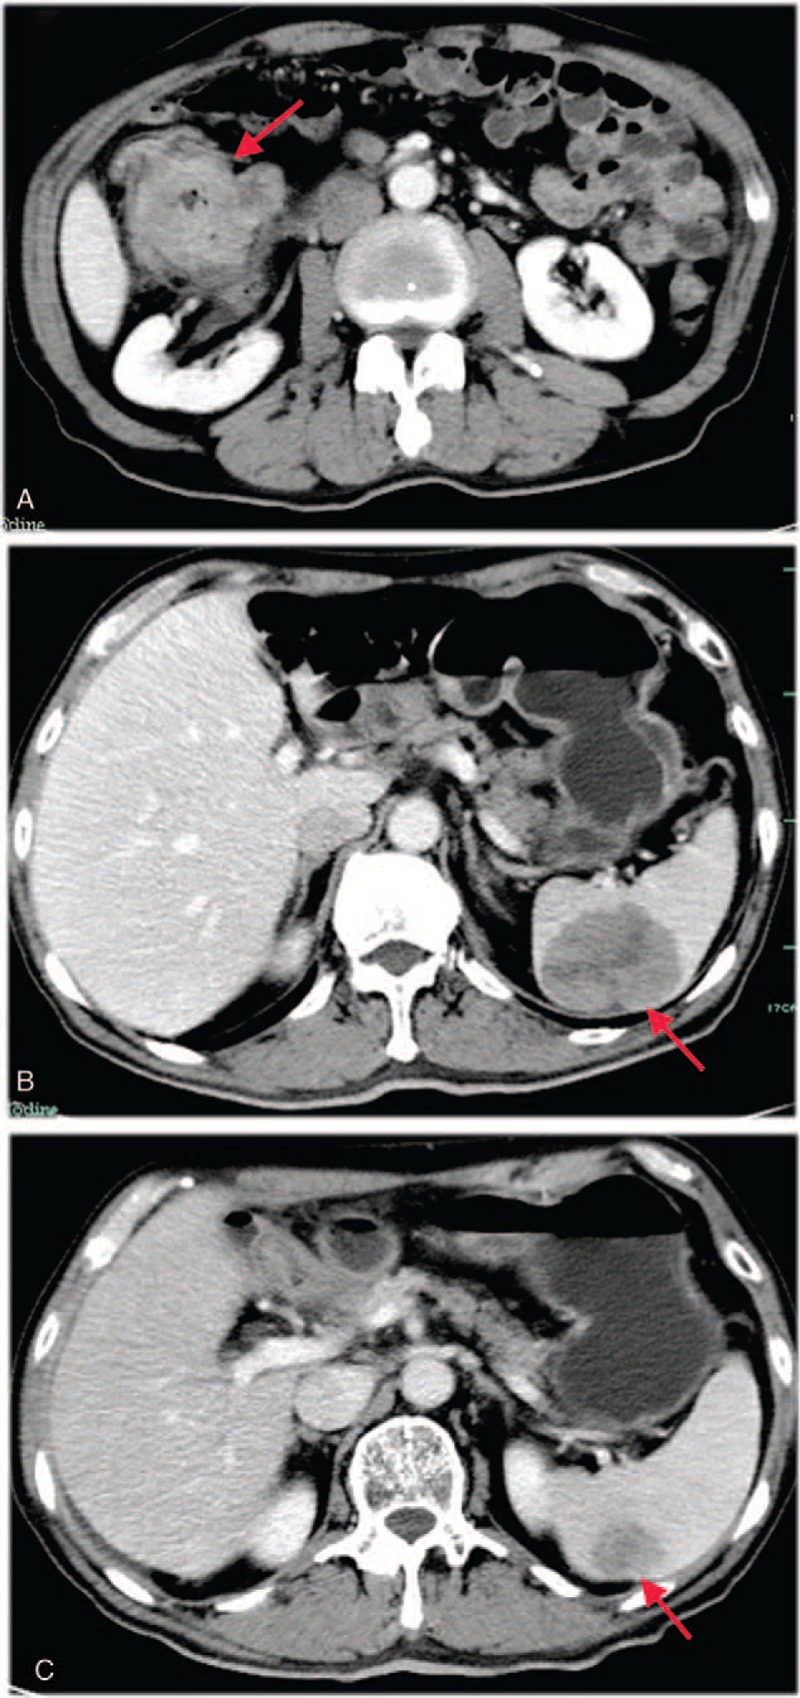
Whole abdominal computed tomography (CT). (A) a heterogeneous enhanced tumor in hepatic flexure; (B) a single low-density lesion of the spleen about 5.7 cm in diameter before primary treatment; (C) the spleen mass about 2.5 cm in diameter after 4 courses chemotherapy with Xelox (capecitabine, oxaliplatin).
